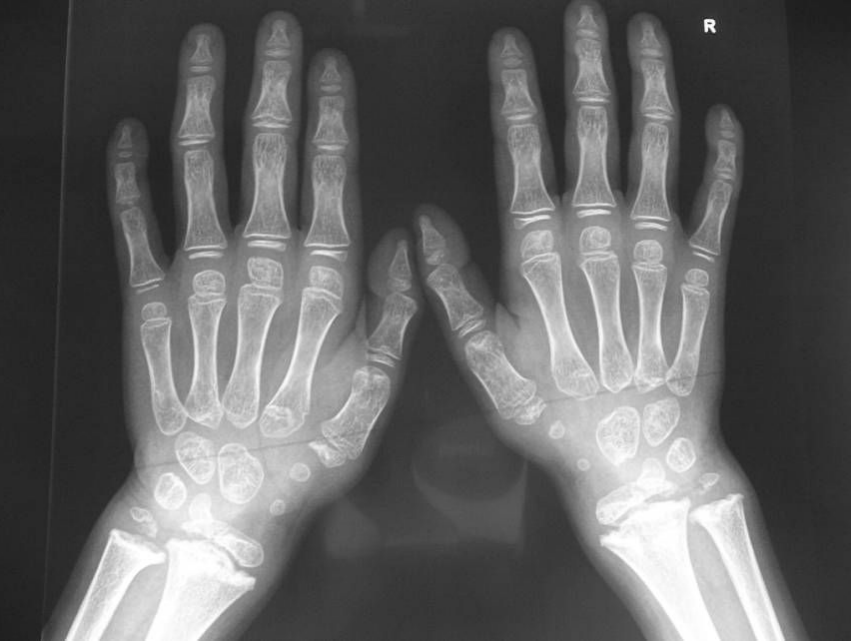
Anteroposterior hand radiograph showed, short-stubby hand with large carpal bones associated with pseudoepiphyses of the second proximal metacarpophalangeal joint.
